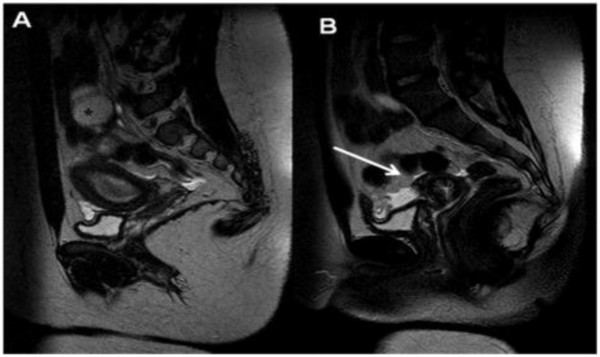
A) Sagittal T2-weighted MR image shows a normal left uterine cavity communicating with a normal cervix (long straight arrow), which in turn communicates with a normal vagina. (B) Right hematosalpinx (asterisk) with a distended non-communicating horn filled with fluid moderately hyperintense on T2 sequences due to haemoglobin degradation products.
